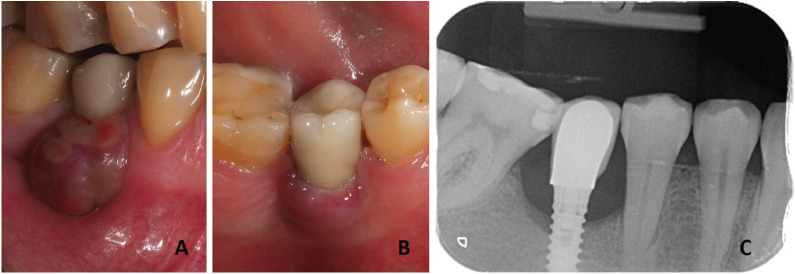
Clinical and radiological characteristics. A) Partially-ulcerated violet-colored sessile-based tumor on the buccal peri-implant mucosa, B) Lingual aspect of the lesion, C) Periapical radiography were marginal bone loss can be observed.
